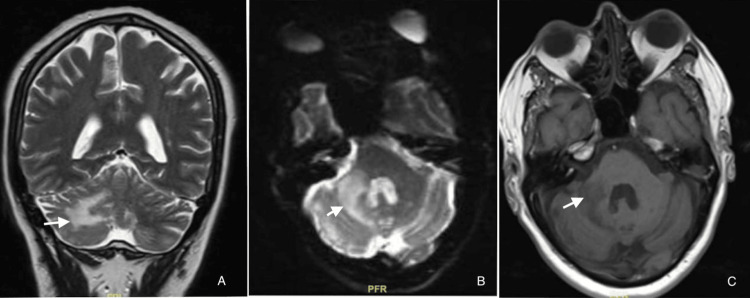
Baseline MRI images (A) MRI brain with a contrast, coronal section in T2 turbo spin echo (TSE) reveals hyperintensity (white arrow) in the right cerebellar hemisphere. (B) MRI brain echo planar 2D (EP2D) differential scan suggestive of hyperintensity (white arrow), in the right cerebellar hemisphere. (C) MRI brain T1-weighted image reveals a hypodense lesion in the right cerebellar hemisphere (white arrow), predominantly involving white matter.
